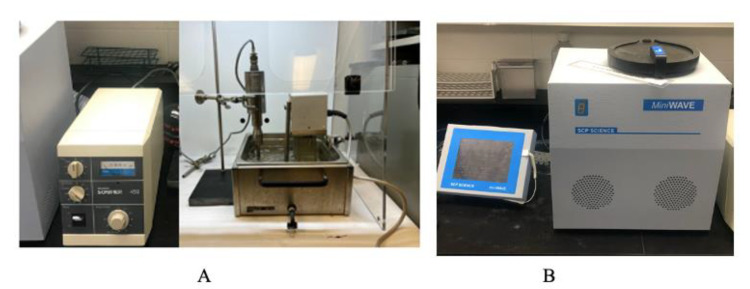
Branson Sonifier 450 ultrasound (A) and mini-wave microwave (B) extraction systems used for the study.
